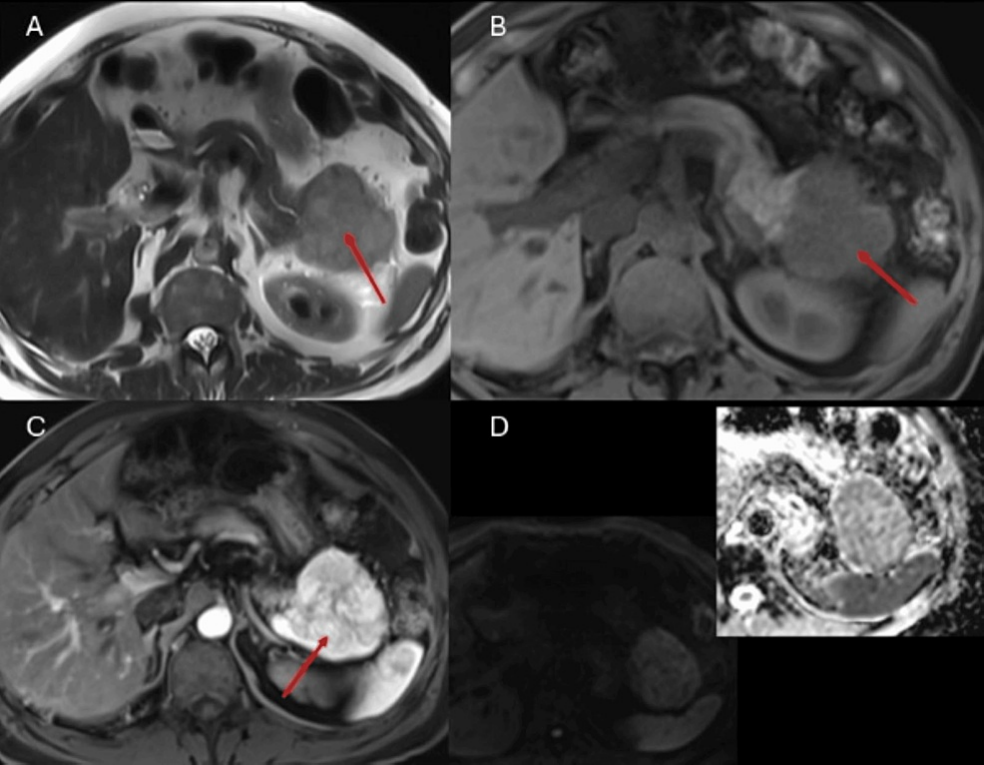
Pancreatic neuroendocrine tumor. Contrast-enhanced magnetic resonance imaging of the abdomen. A: T2-weighted sequence in axial section; B: T1-weighted sequence without contrast in axial section; C: T1-weighted sequence after gadolinium administration in axial section. D: diffusion sequences. A solid, rounded lesion is observed, located in the tail of the pancreas, it is hypointense on the T1 and T2 sequences with respect to the pancreatic parenchyma (red arrows) and has mild diffusion restriction. Findings compatible with pancreatic neuroendocrine tumor.
